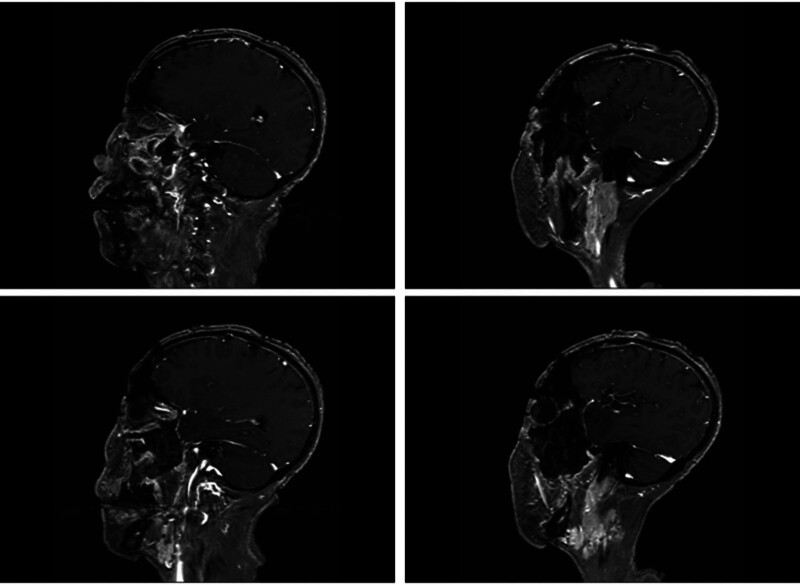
Patient postoperative MRI-enhanced scanning cross-sectional T1 phase. MRI = magnetic resonance imaging.
